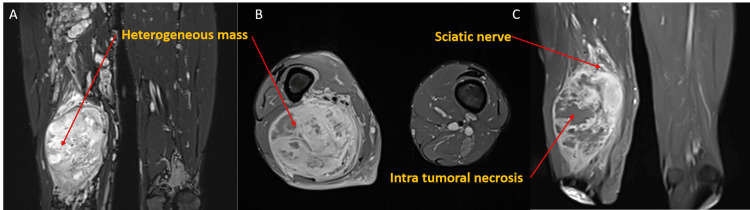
MRI of the thigh showing a malignant peripheral nerve sheath tumor. (A) Coronal fat-suppressed T2-weighted image demonstrating a large heterogeneous soft tissue mass of the thigh with marked craniocaudal extension. (B) Axial post-contrast fat-suppressed T1-weighted image showing heterogeneous enhancement (C) with internal non-enhancing areas suggestive of necrosis. Long-axis post-contrast image further depicting the lesion extent and internal heterogeneity, with the mass appearing in close contact with the sciatic nerve.
